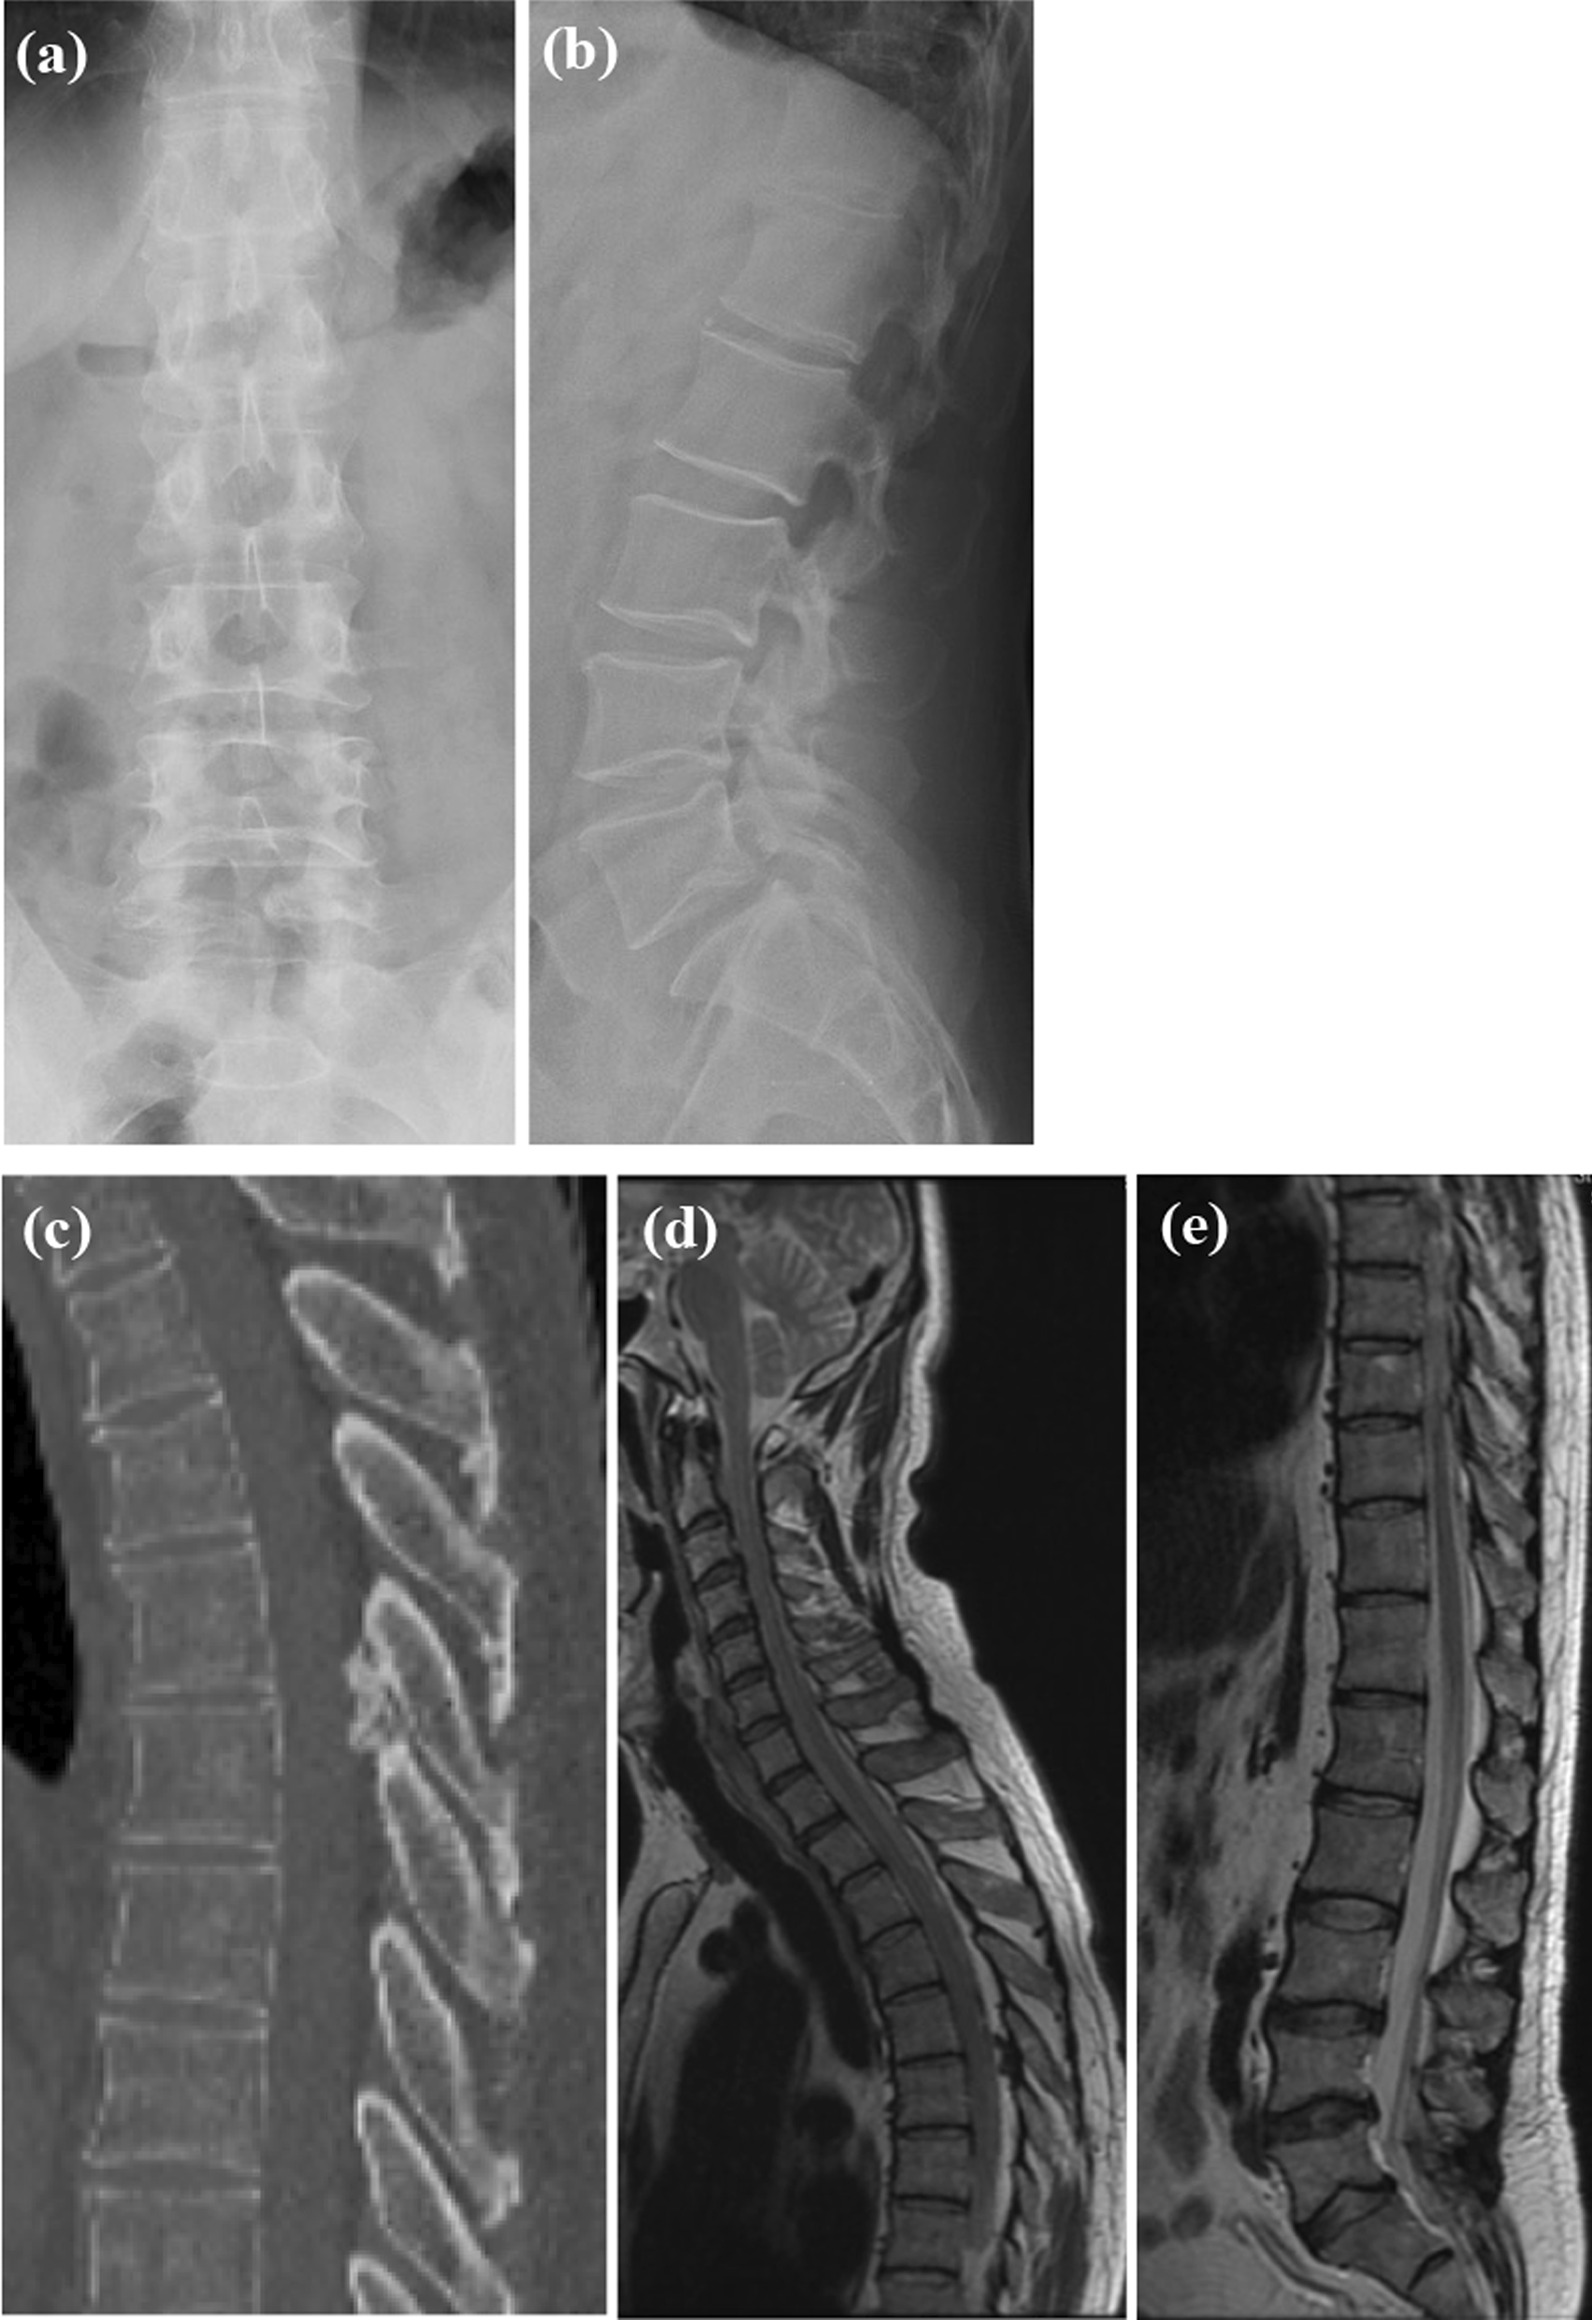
Imaging results of the injury. Anteroposterior view (a) and lateral view (b) of lumbar plain radiography performed after the injury. Sagittal view (c) of the thoracic spine CT and T2-weighted MRI of the cervical spine (d) and lumbar spine (e) 1 month after the injury. Degenerative changes in the lumbar spine and ossification of the ligamentum flavum at T5/6 were noted. However, there were no signs of significant spinal cord compression
